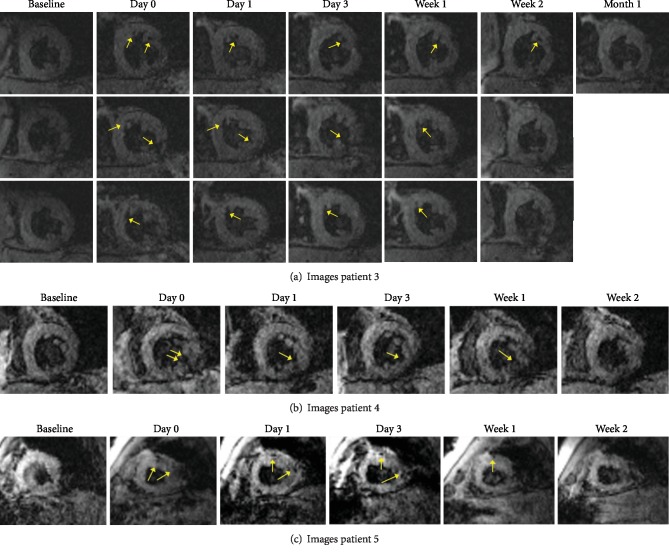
MRI images of patients 3–5. T2∗ images from patient 1 are in the main text. Images from patient 2 are not shown due to cardiac tamponade. The yellow arrows point to hypointense areas in the images suspected to point out injected USPIO-labeled MSCs.
